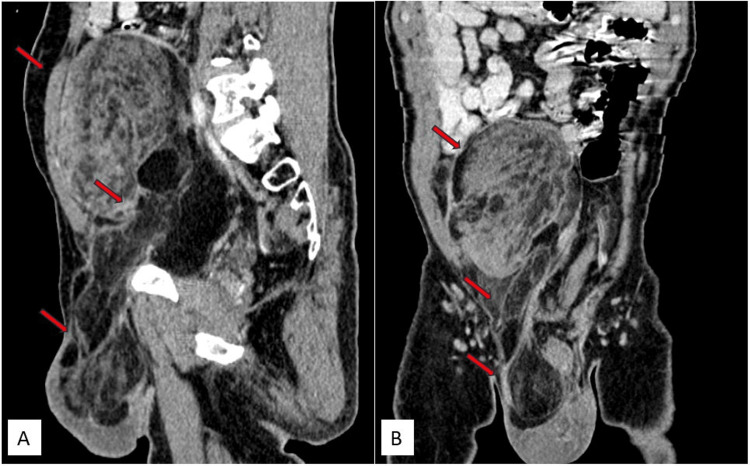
Contrast CT images (sagittal and coronal) depict a solid mass of heterogeneous density, showing both lipomatous and non-lipomatous components, in the retroperitoneum. It extends inferiorly into the pelvis, right inguinal region, and right scrotal sac up to the upper pole of the right testis. The solid component exhibits heterogeneous enhancement. Contrast CT images reveal a mass of heterogeneous density in the retroperitoneum extending inferiorly into the pelvis, right inguinal region, and right scrotal sac, reaching up to the upper pole of the right testis in sagittal (red arrows) (A) and coronal (red arrows) (B) sections. CT: computed tomography
